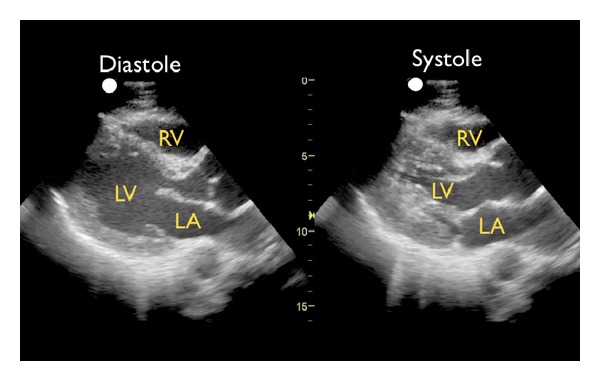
Good left ventricular contractility, parasternal long axis view. RV: right ventricle, LV: left ventricle, LA: left atrium.
